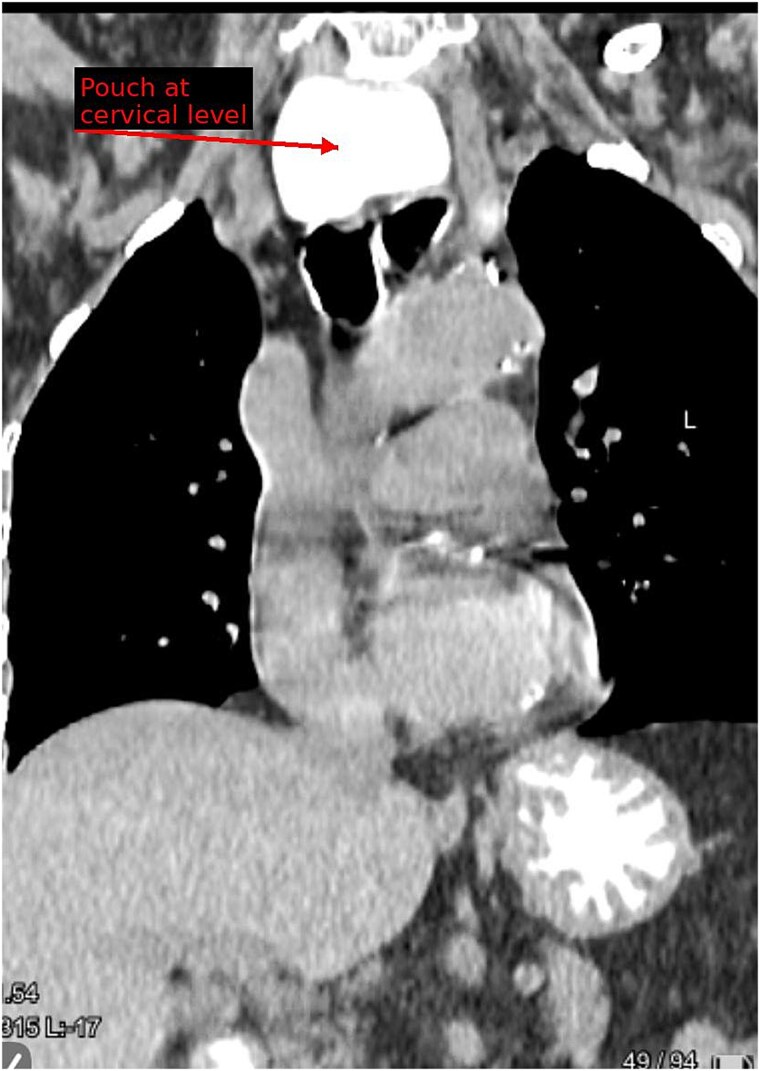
Contrast-enhanced CT (coronal reconstruction) demonstrating the cervical-level pouch and its craniocaudal extension.
